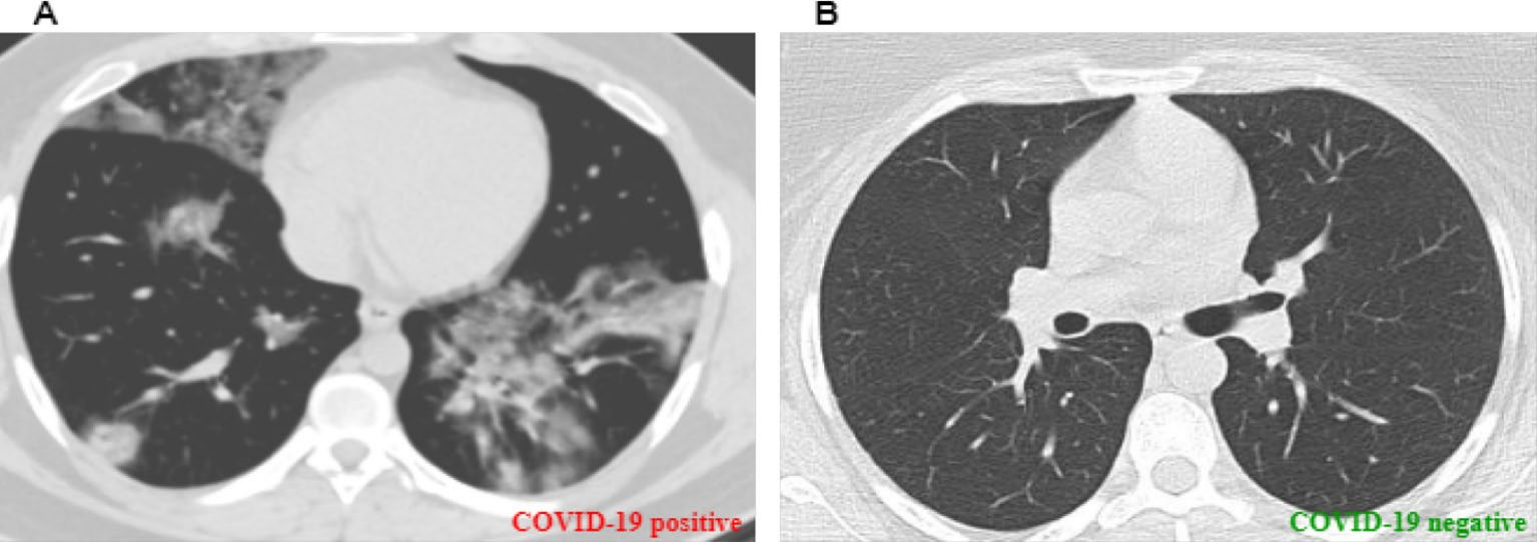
CT scan images of COVID-19 positive and negative.
